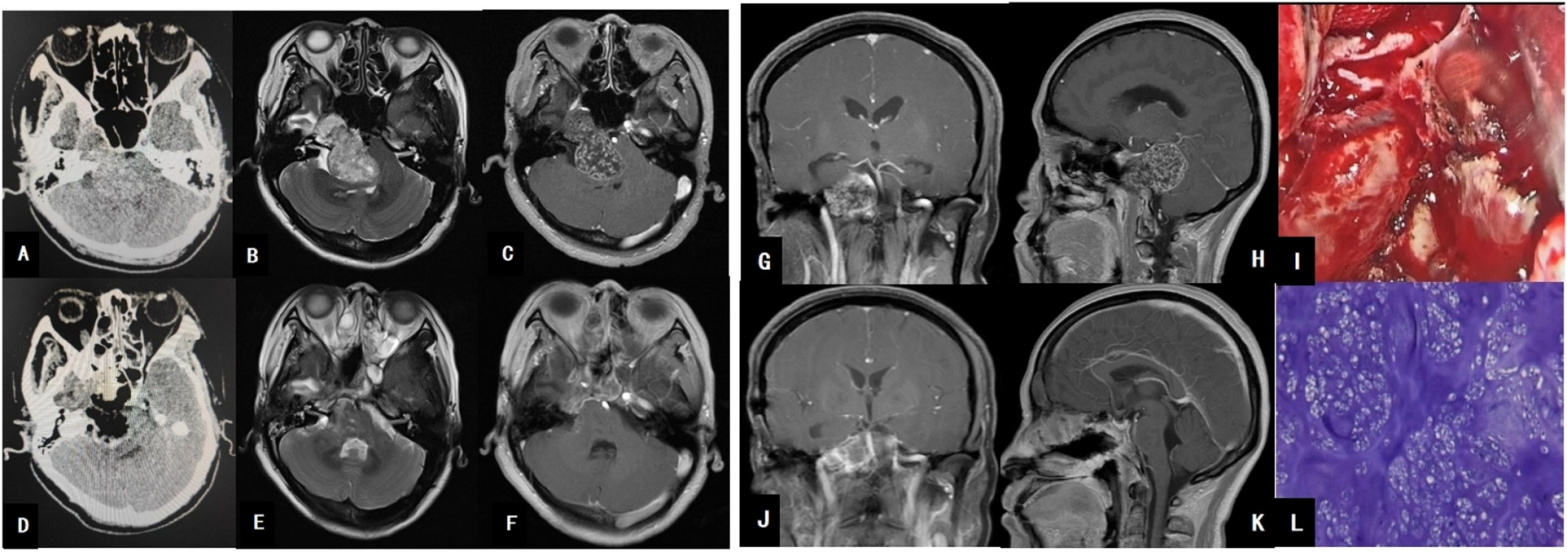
(A) Brain CT shows a large hyperdense mass in the right middle and posterior cranial fossa. (B) MRI T2 reveals a large hyperintense mass in the middle and posterior cranial fossa. (C) MRI T1 axial contrast-enhanced image demonstrates heterogeneous enhancement of the large tumor, closely related to the petrous segment of the internal carotid artery. (G) MRI T1 coronal contrast-enhanced image shows the tumor’s close relationship with the basilar artery. (H) MRI T1 sagittal contrast-enhanced image reveals tumor compression on the cerebellum and brainstem. (I) Intraoperative view of tumor resection. (D) Postoperative CT changes after tumor resection. (E,F,J,K) Postoperative MRI changes, indicating complete tumor resection. (L) Pathological image of the tumor.
